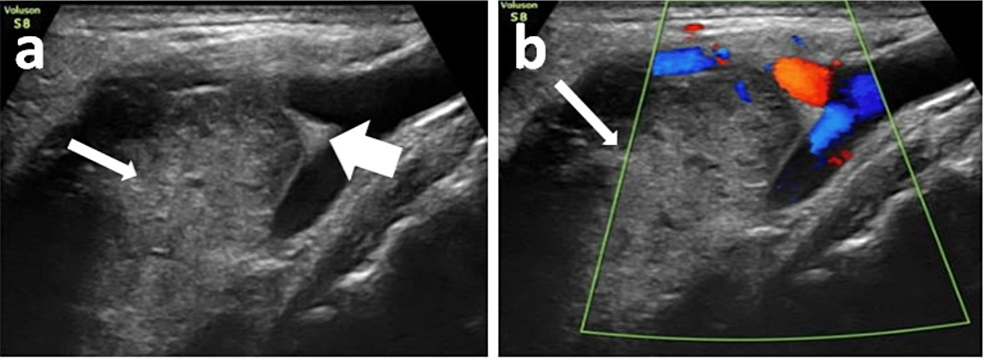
Ultrasound images of the neck demonstrating a lesion at the bifurcation of the common carotid artery (CCA). (a) USG neck (longitudinal orientation) showing a well-defined relatively heterogeneous iso- to hyperechoic to muscle lesion (long white arrow) in the region of bifurcation of CCA, causing splaying of ECA and ICA (short white arrow). (b) The lesion (long white arrow) does not show internal vascularity (Probe marker: Voluson S8; GE Healthcare, Chicago, USA). ECA: external carotid artery; ICA: internal carotid artery
